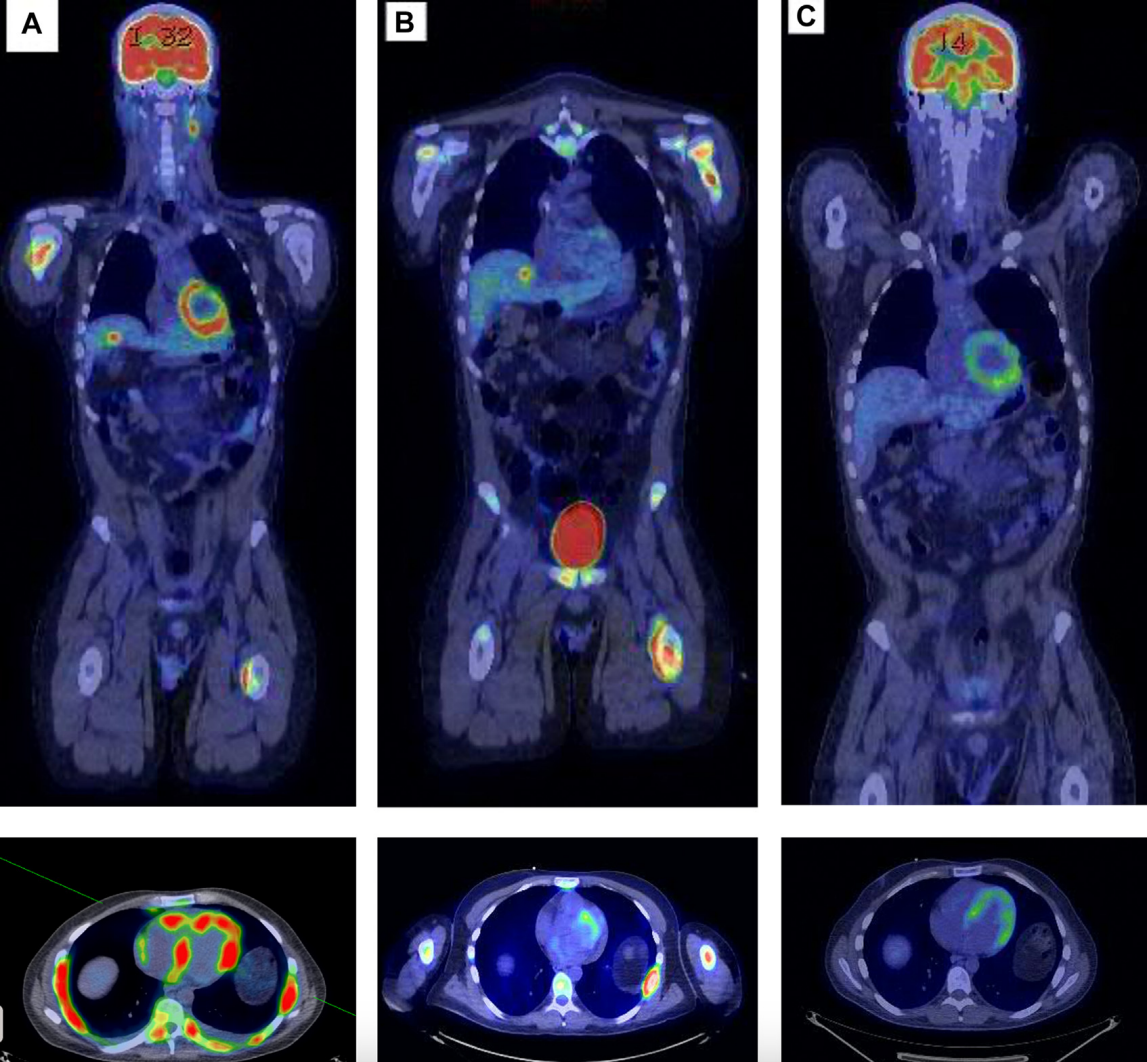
Serial PET-CT Scans Showing Lymphoma Involving the Myocardium in Patient 1 (A) Positron emission tomography–computed tomography (PET-CT) scan of Patient 1 at baseline shows nodular hypermetabolic activity in the myocardium, bones, and liver. (B) Interval PET-CT scan after bridging therapy shows improvement in fluorodeoxyglucose uptake in the myocardium. (C) At 1 month after chimeric antigen receptor T-cell therapy, PET-CT scan shows a near complete metabolic response systemically (except for physiological myocardial uptake).
